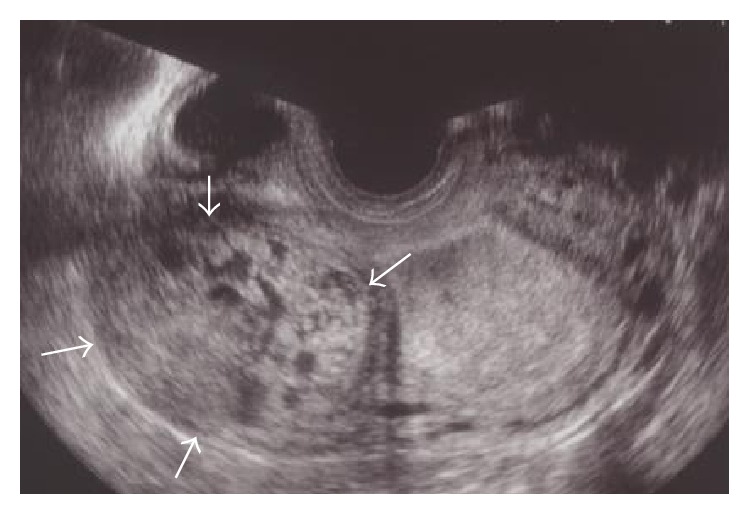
Transvaginal ultrasonographic image at 8 weeks of gestation. A hyperechoic mass (5 cm) in the right cornu containing multiple vesicles (arrows).
